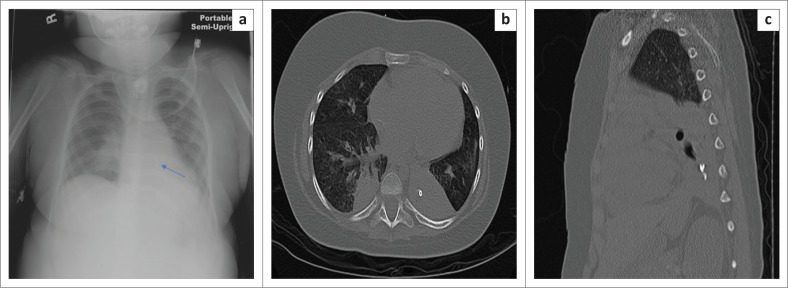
Images of a 6-year-old child with severe anoxic encephalopathy who presented with a history of teeth aspiration. The aspirated teeth are barely visible (arrow) on the chest radiograph (a). Multi-detector computed tomography axial (b) and sagittal reformatted (c) images confirmed the location of the aspirated teeth in the left lower lobe bronchus.
